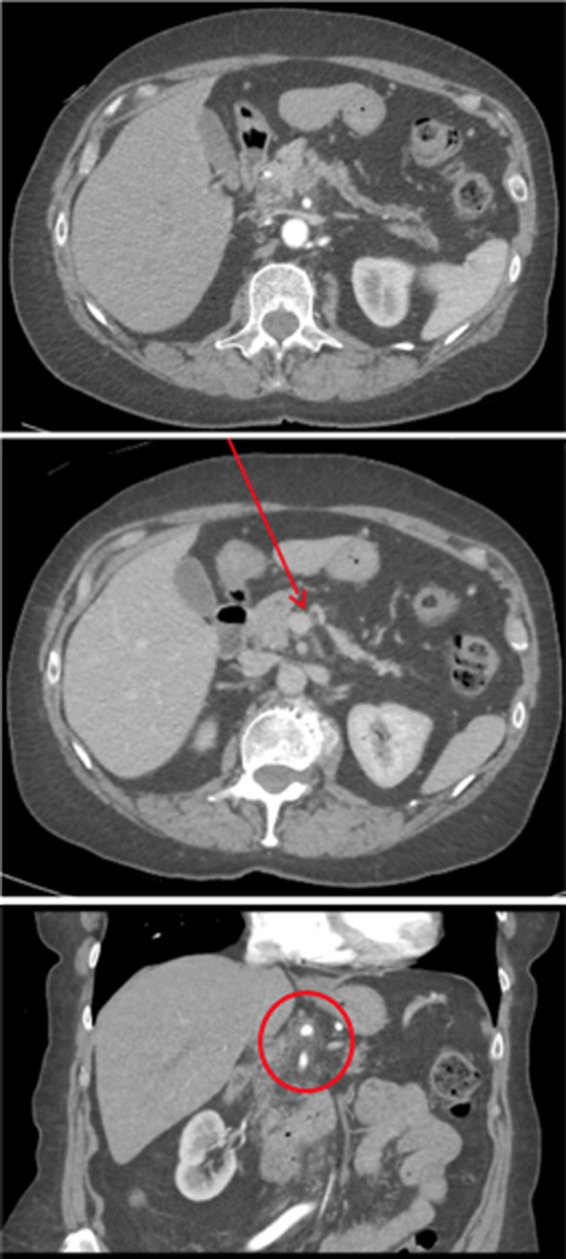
CT scan post multidrug neoadjuvant chemotherapy with a poorly defined infiltrative pancreatic body mass measuring ∼25 × 15 mm (top) with ill-defined soft tissue encasing the proximal splenic artery, common hepatic artery, distal celiac axis, and superior mesenteric artery (bottom). The mass (arrow) also abuts the portal vein (middle) and superior mesenteric vein.
